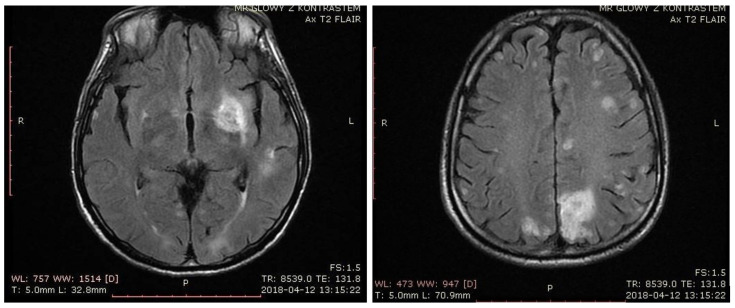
MRI performed after three weeks of treatment showing reduced perilesional edema and sharper borders in some of the lesions.
